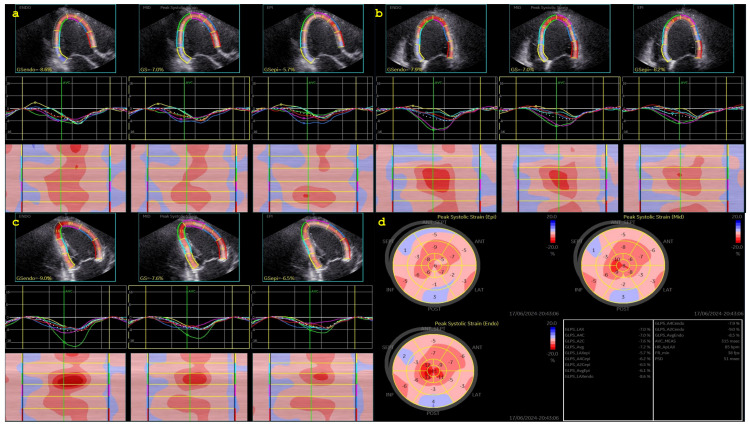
Layer-specific longitudinal strain analysis. (a) Apical 3-chamber view. (b) Apical 4-chamber view. (c) Apical three-chamber view. (d) Bulls-eye display of layers of strain.
